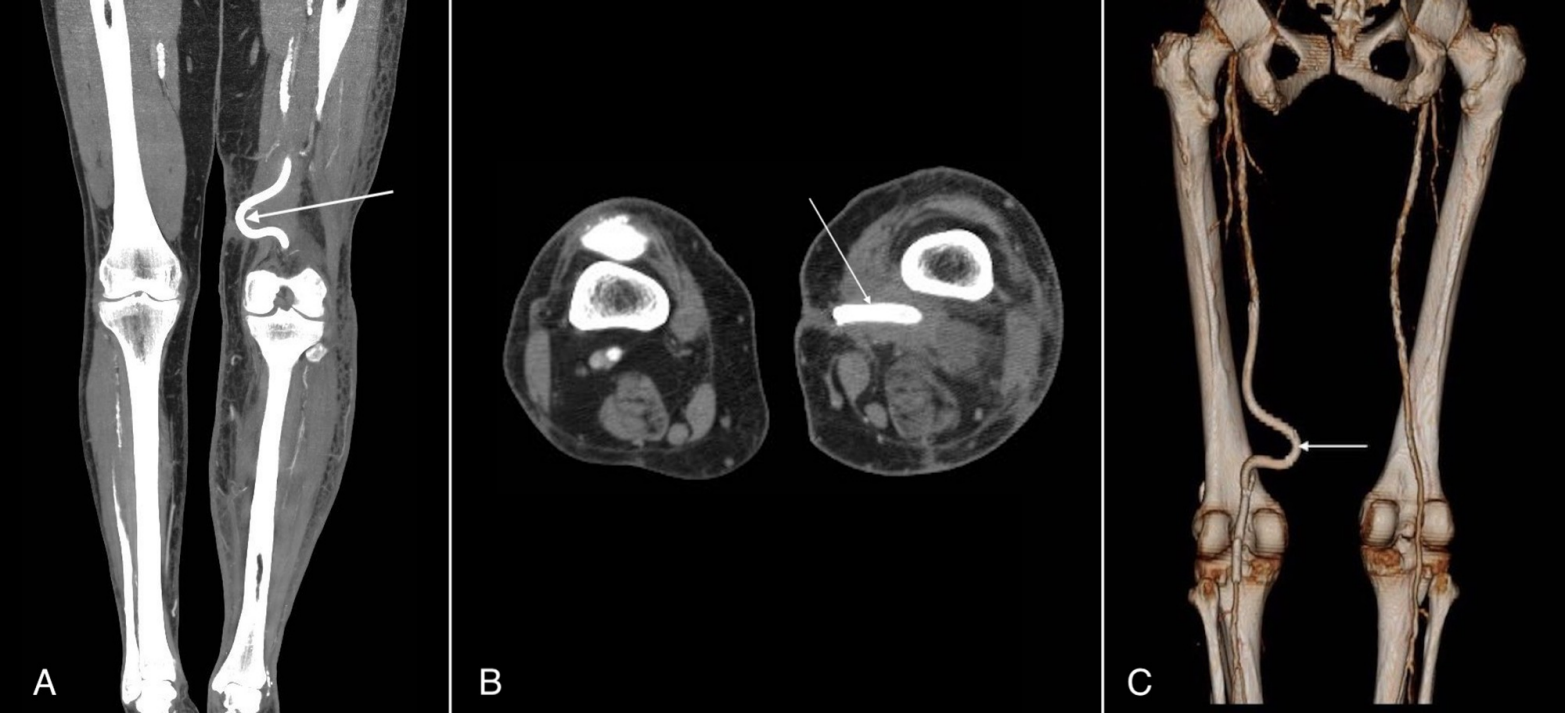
(A, B, C) CT one year after the initial perforation and stenting shows severely deviated and tortuous stent-graft (arrows) in the left adductor canal, bulging into subcutaneous layers merely 7 mm from the skin’s surface; without any pseudoaneurysm. CT - Computed tomography
